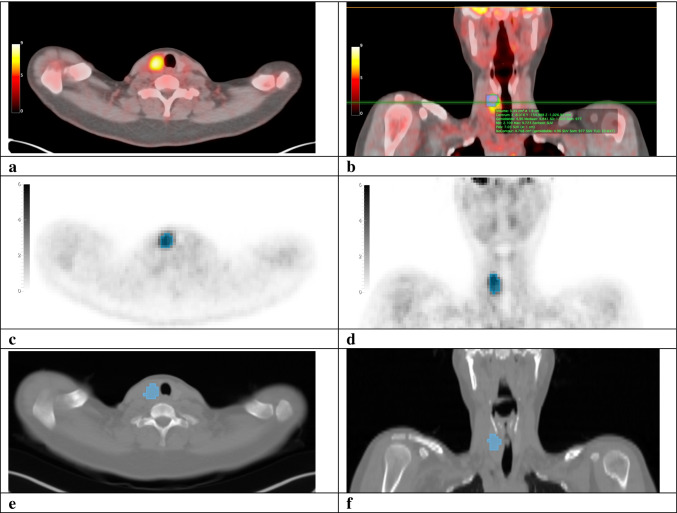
Quantitative [^18^F]FDG-PET/CT assessment and delineation of the VOI for radiomic analysis. Transverse and coronal [^18^F]FDG-PET/CT (a, b), maximum intensity projection (MIP) (c, d) and low-dose CT (e, f) images of a patient with a solitary, 30 mm Bethesda III thyroid nodule in the right lobe. Visual assessment (a) of the [^18^F]FDG-PET/CT showed an [^18^F]FDG-positive index nodule. Quantitative assessment (b) demonstrated a SUV_max_ of 9.7 g/mL and SUV_peak_ of 7.0 g/mL of the index nodule, and a SUV_max_ of 1.6 g/mL in the background of surrounding normal thyroid tissue. Consequently, the SUV_max_-ratio and SUV_peak_-ratio were 6.1 (9.7/1.6) and 4.4 (7.0/1.6), respectively. For radiomic analysis, VOIs were delineated on the [^18^F]FDG-PET scans using an isocontour that applies a threshold of 50% of the SUV_peak_, corrected for local background (c, d) [29]. Boxing was applied to exclude [^18^F]FDG-positive tissue surrounding the index nodule and ldCT images were used as a visual reference (e, f). VOIs delineated on the PET images were resampled with a nearest neighbour algorithm to derive the ldCT VOIs
